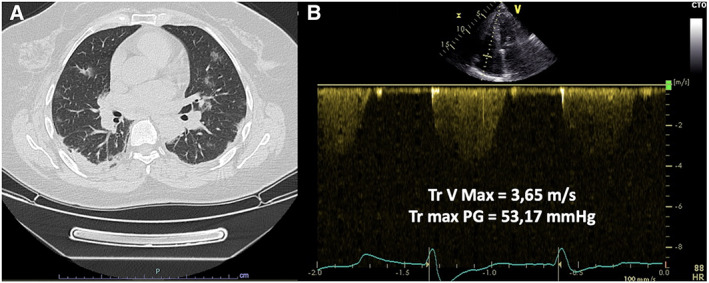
(A) TTE continuous wave Doppler on tricuspid valve suggestive for severe pulmonary hypertension. (B) Chest computed tomography revealed bilateral ground‐glass opacifications and smooth interlobular and intralobular septal thickening suggestive for interstitial pneumonia. PG, peak gradient; TTE, transthoracic echocardiography.
